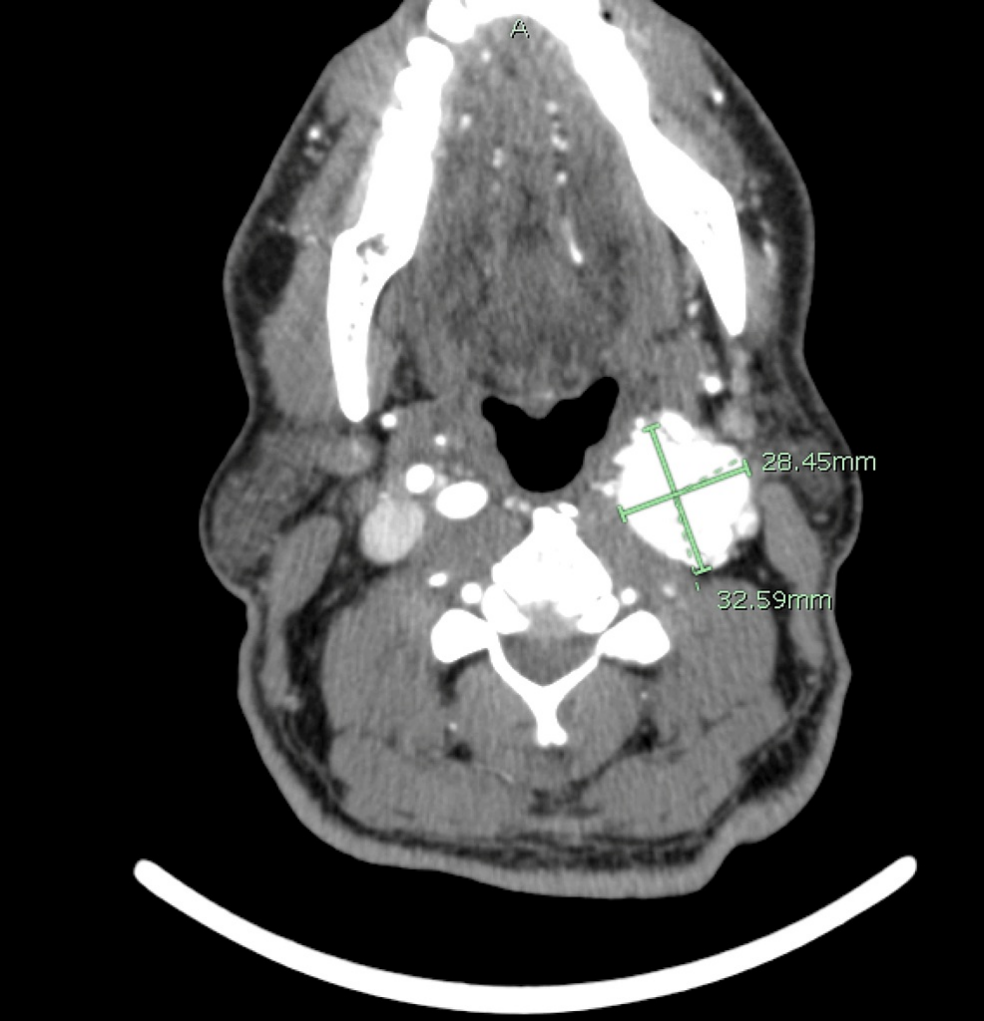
A transverse head and neck CTA showing a left neck mass measuring 3.3 x 2.8 cm in anterior-posterior and lateral measurements
